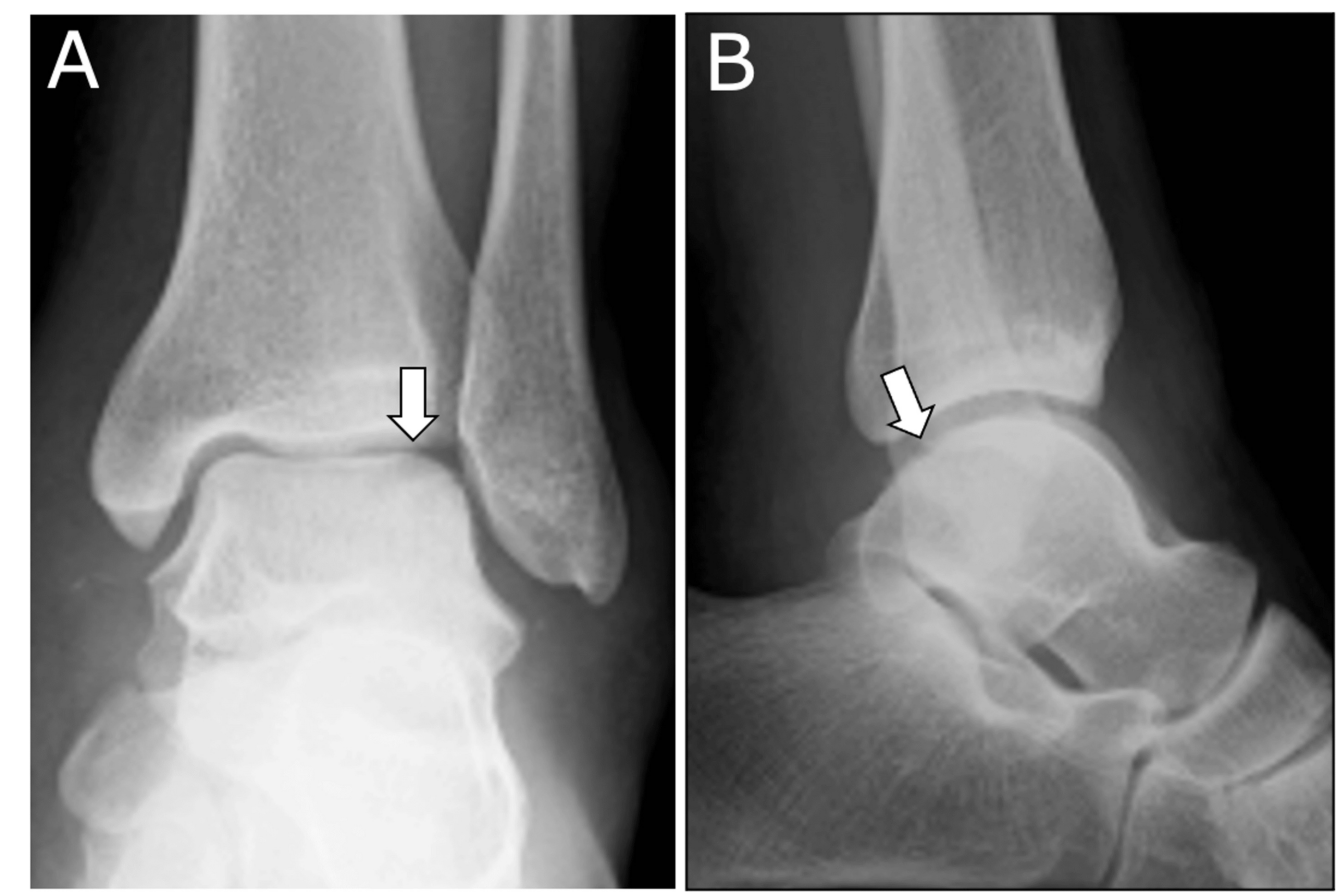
Preoperative plain radiographs A: The anteroposterior view demonstrates a radiolucent area in the lateral talar dome (arrow). B: The lateral view reveals an irregular contour of the posterior talar dome (arrow).
